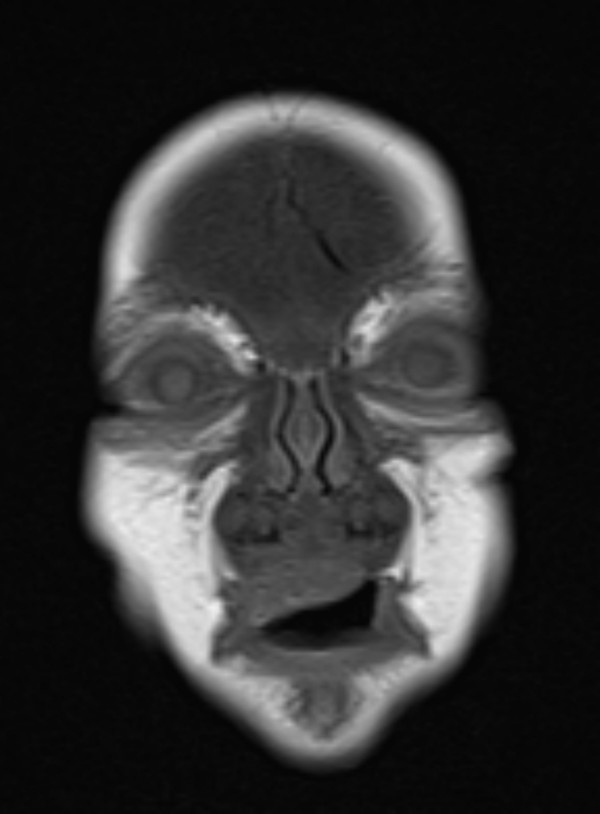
Coronal MRI imaging showed significant calvarial/facial and mandibular hyperostosis.
